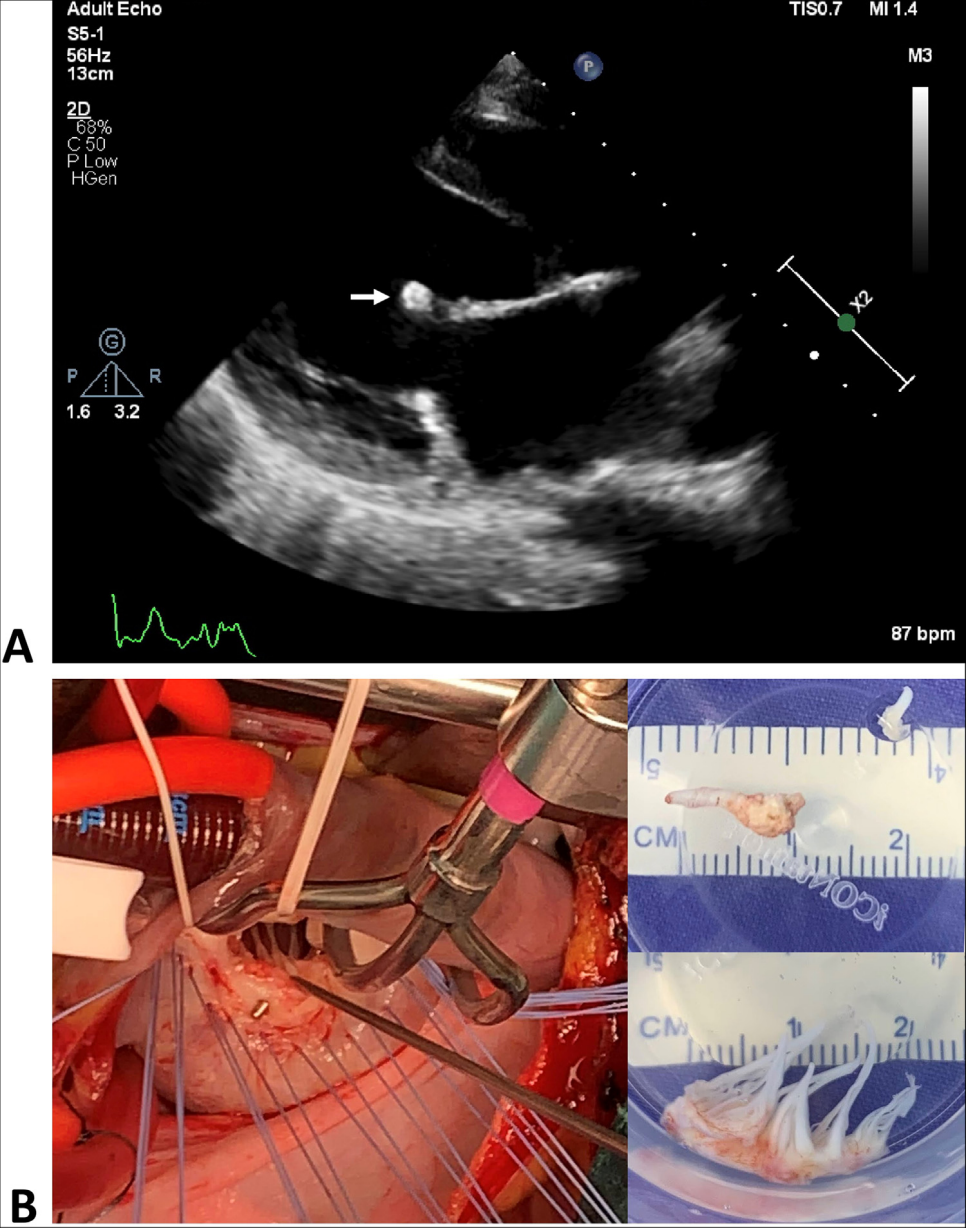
(a) A transthoracic echocardiogram showing a 0.9 cm x 0.7 cm oscillating mass on the anterior mitral leaflet (white arrow). (b) Intraoperative findings demonstrating the perforated P2 cusp of the mitral valve (left), two fragments of tissue from the mitral valve, measuring 1.2×0.5×0.2 cm on the fragment with vegetation (above), and 2.5×1.5×1.5 cm (below).
